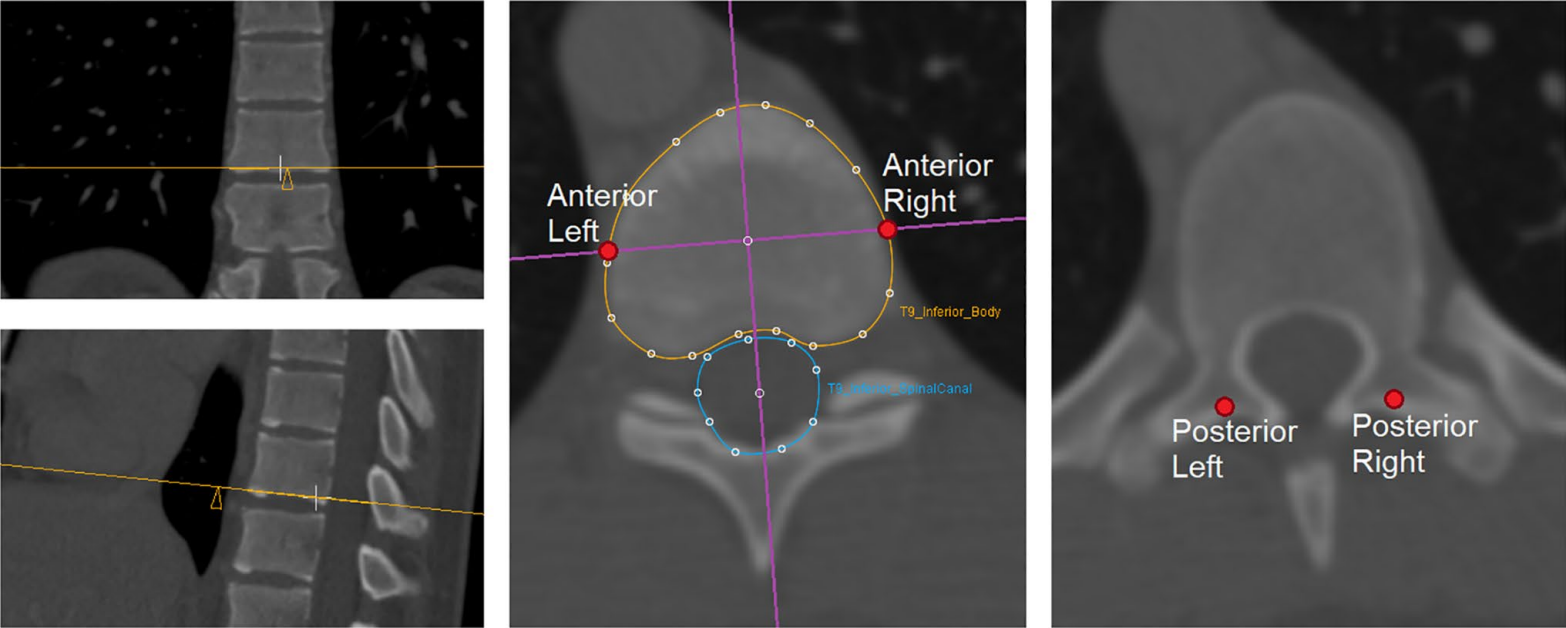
In the CT analysis software the upper and lower endplates of all vertebral bodies in the right-convex thoracic curves were segmented in the ‘true’ transverse plane. The spinal canal was also segmented to calculate the mid-sagittal axis, which intersects with the centroid of the endplate and the centroid of the canal. Perpendicular to the mid-sagittal axis at the centroid, the left and right point per was calculated. The mid-point between the upper and lower left point was calculated to retrieve the left anterior column point for each vertebrae, and similarly for the right side. Finally, on the transverse plane, where the intersections of the laminae and transverse process were visible, this was segmented to retrieve the left and right posterior column point per vertebrae
